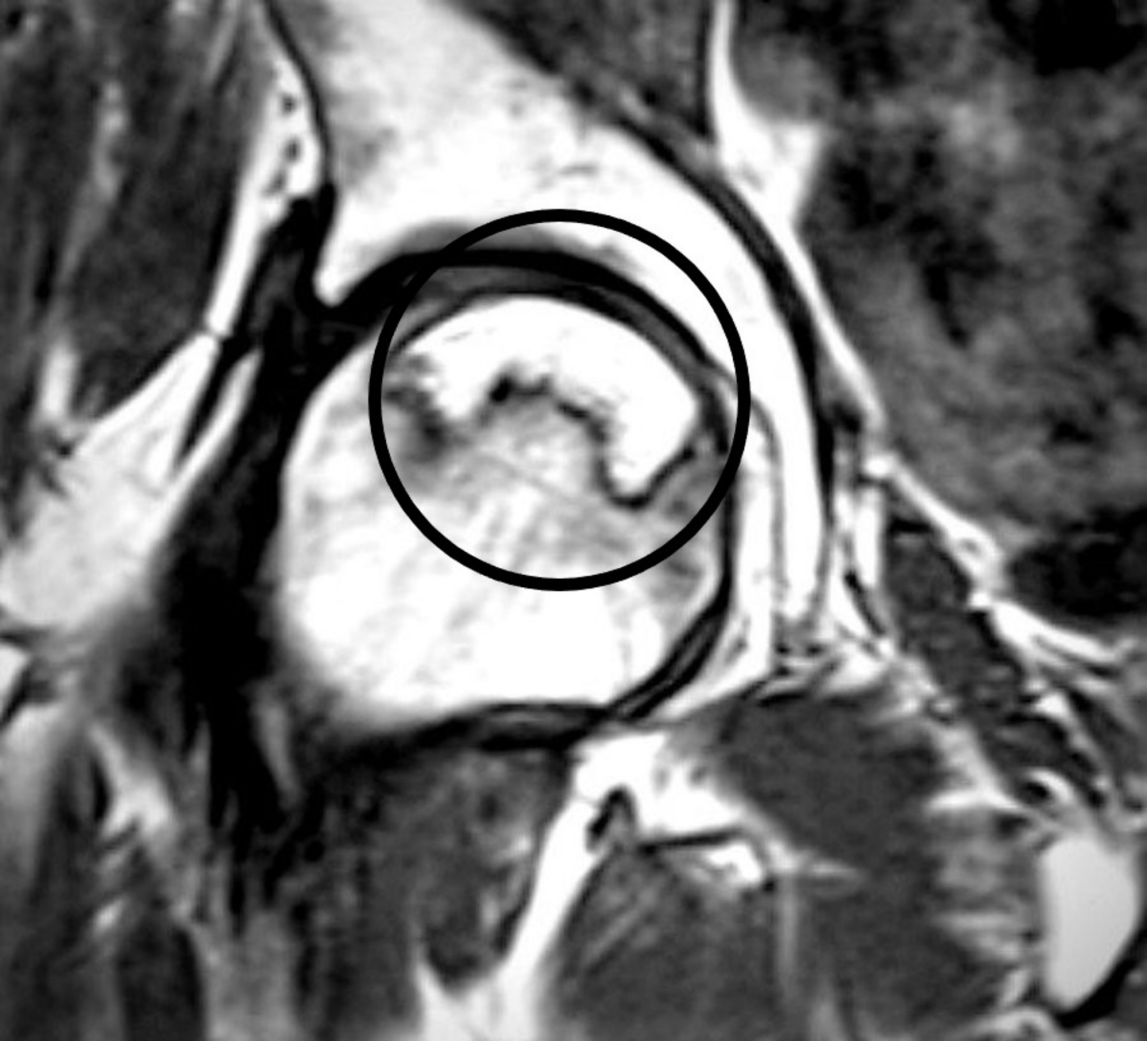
MRI left hip T1 T1 MRI image encircled, showing osteonecrosis in the femoral headband-like lesion. MRI: Magnetic resonance imaging
